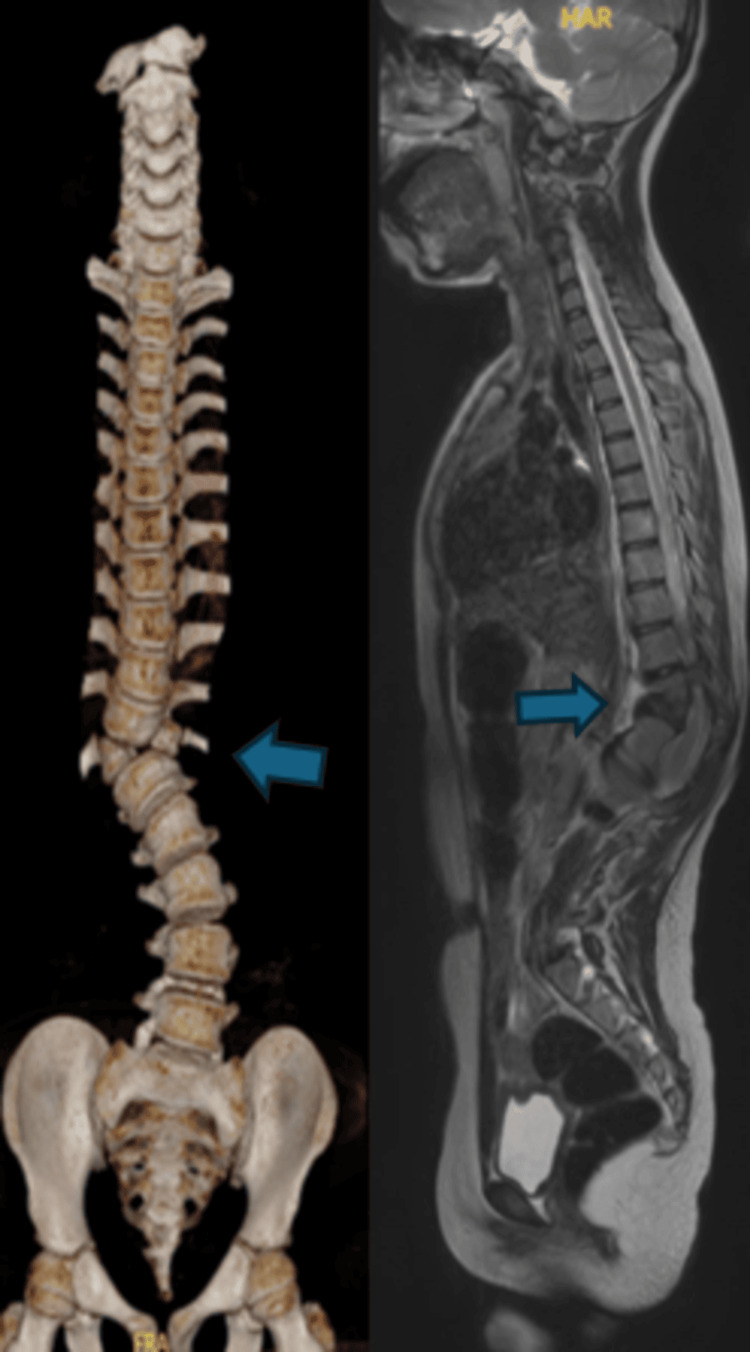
Three-dimensional computed tomography image (left) of the whole spine in coronal view showing lumbar levoscoliosis with T12 hemivertebra. Magnetic resonance imaging (right) of the whole spine in sagittal view showing thoracolumbar dextroscoliosis with butterfly vertebrae of T12.
